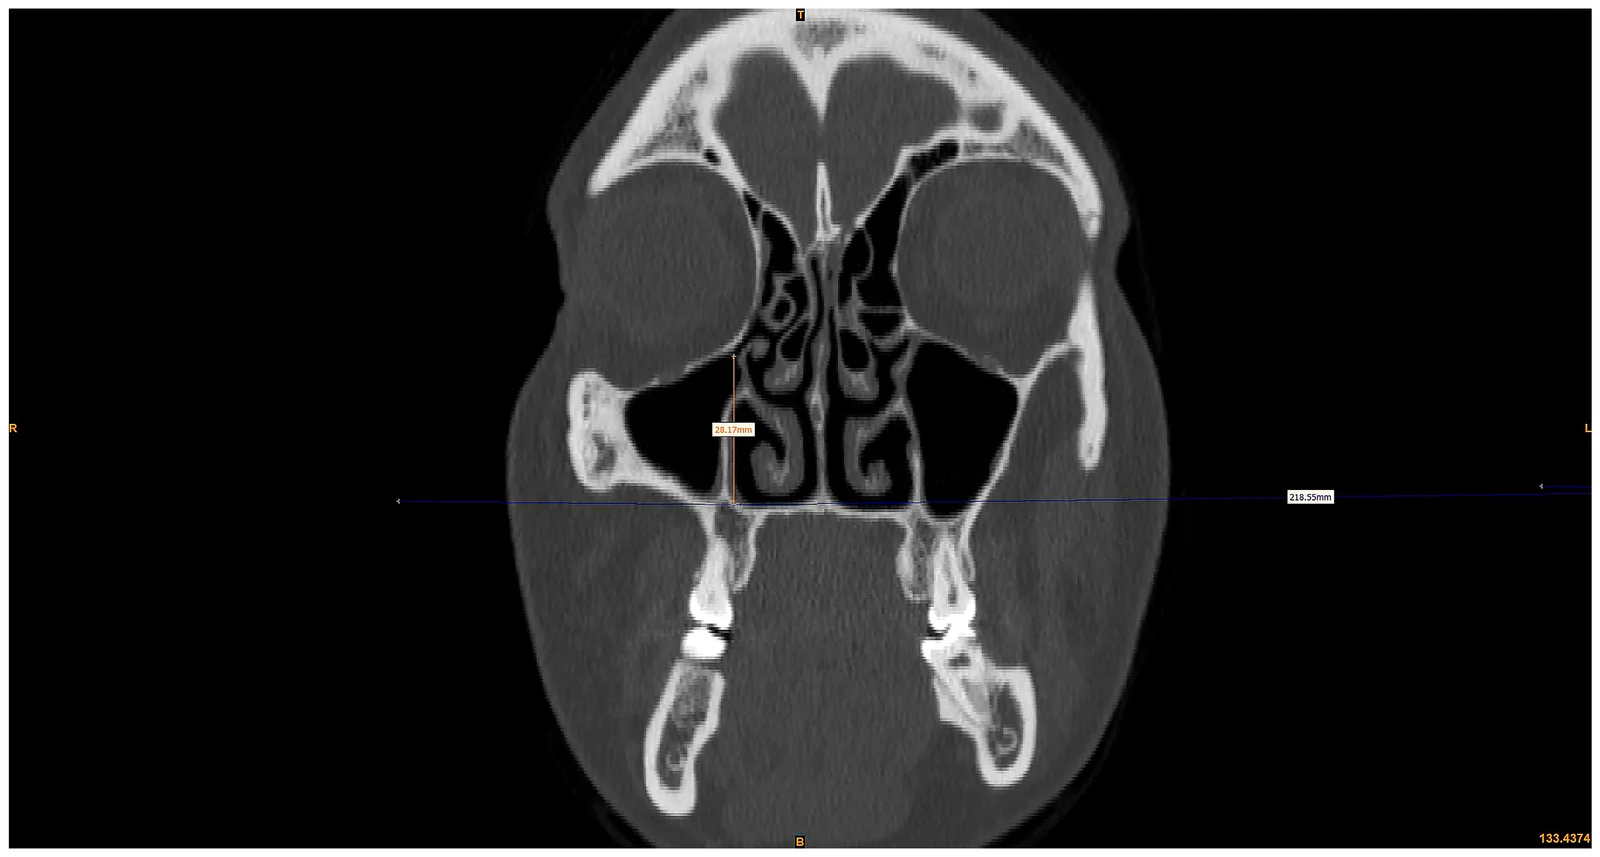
Measurement of the ostium-to-sinus-floor distance (OSFD) on the coronal CT image. The linear distance was measured from the inferior margin of the natural maxillary ostium to the lowest point of the maxillary sinus floor on the coronal section demonstrating the natural ostium most clearly. Abbreviations: R, right; L, left; T, top; B, base.
